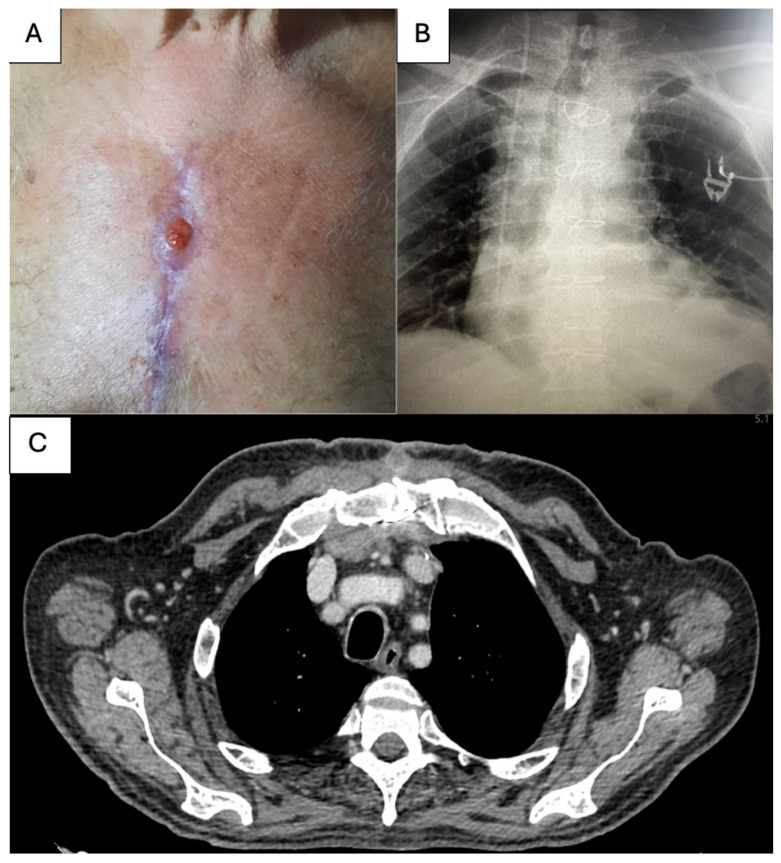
(A) Clinical presentation of SCF; (B) Chest XR at presentation; (C) Chest CT aspect at presentation.
